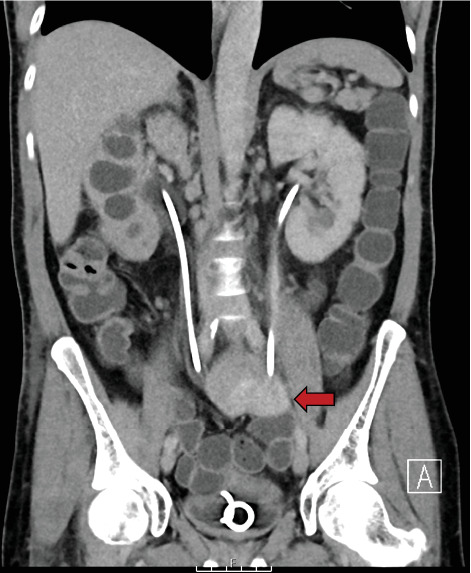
Initial CT urogram (coronal plane). A further portion of the small bowel with contrast extravasation can be seen. The left perinephric stranding is also visible in this image.
